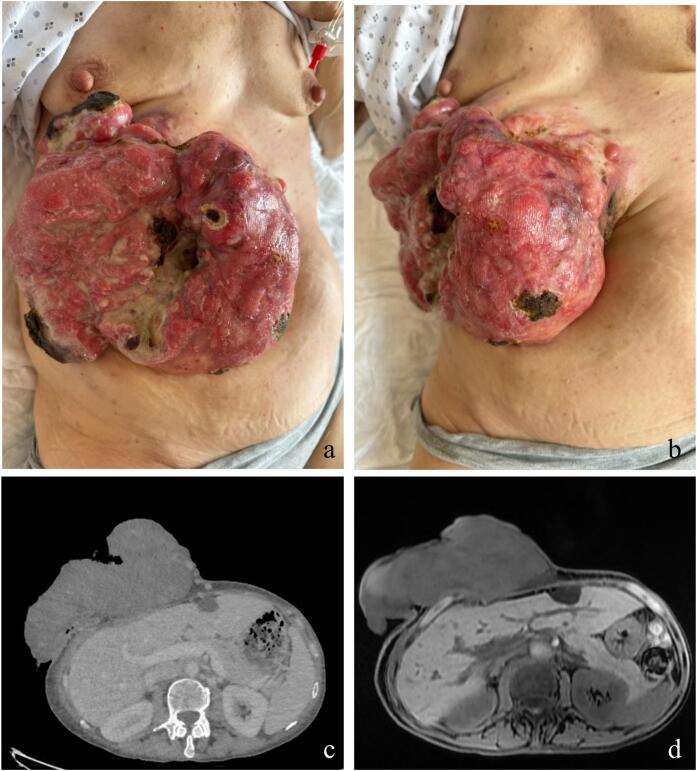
Pre-operative considerations of the large abdominal DFSP. a. Top view photograph of the voluminous abdominal mass. b. Photograph of the ulcerated nodular abdominal mass, showing the tumor base located along the midline. c. CT scan showing a lesion invading the upper portion of the rectus abdominis muscle and hepatic scalloping at the junction of segments IV and V, two months prior to surgery. d. MRI performed two months prior to surgery, showing a tumor measuring 20 cm in transverse diameter, 11 cm in maximal depth, and 19 cm in vertical height on the selected view. The mass was in close contact with the liver and the right 6th to 10th chondrosternal cartilages, without clear evidence of invasion.
